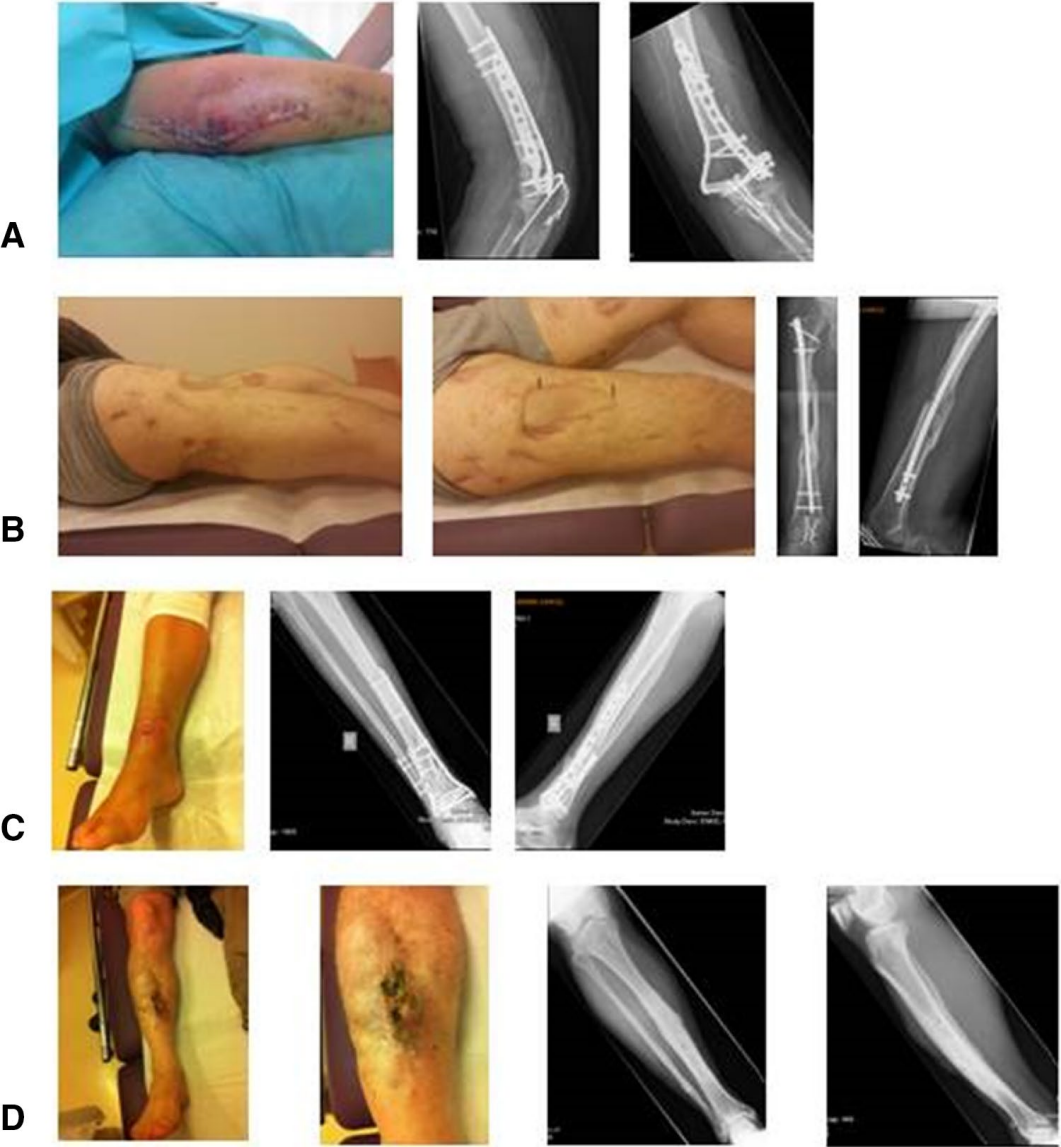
Patient-based clinical scenarios. Patient A: A 58-year-old healthy man underwent an open reduction and internal fixation (ORIF) of a comminuted intra-articular distal humerus fracture 1 week ago. The postoperative X-Ray showed an adequate fracture reduction and good position of the osteosynthetic material. After 1 week, a wound infection was diagnosed and it was decided to bring the patient back to theatre for a wound washout. His CRP is 68 mg/l, white cell count 11.5 × 10^9^/l. Patient B: A 23-year-old healthy man underwent intramedullary nailing for a Gustillo grade 3B open comminuted femur fracture 1 year ago. The initial stabilization was followed by multiple wound debridements, changing of Vacuum Assisted Closure (VAC) dressings and finally split skin grafting of the wound. During the repeated VAC changes, the nail was palpable in the wound. The patient’s main complaint is pain around the fracture site over the last few months. On examination, there is no wound breakdown. His CRP is 27 mg/l, white cell count 6.5 × 10^9^/l, and ESR 48 mm/hr. Patient C: A 44-year-old healthy woman underwent ORIF of a closed comminuted distal tibia fracture 5 months ago. She is referred because the operation wound broke down 2 weeks postoperatively and has not healed since. Her CRP is 3.3 mg/l, white cell count 8.1 × 10^9^/l. Patient D: A 49-year-old healthy man underwent multiple operations because of an open fracture of his right tibia and fibula 30 years ago. Although the treatment was complicated by a deep surgical site infection, bone healing was eventually achieved and all metal implants were removed a few years after his last operation. The wound settled down until 18 months ago when an unstable crust developed in the scar. His CRP is 5.9 mg/l, white cell count 8.9 × 10^9^/l. Question after each case description: a) would you order a conventional X-Ray? (Note: X-rays are only provided if the responder selected ‘yes’). b) Would you request further imaging? If yes: please select preferred imaging modality (more than one answer possible, please see “materials and methods” for details)
